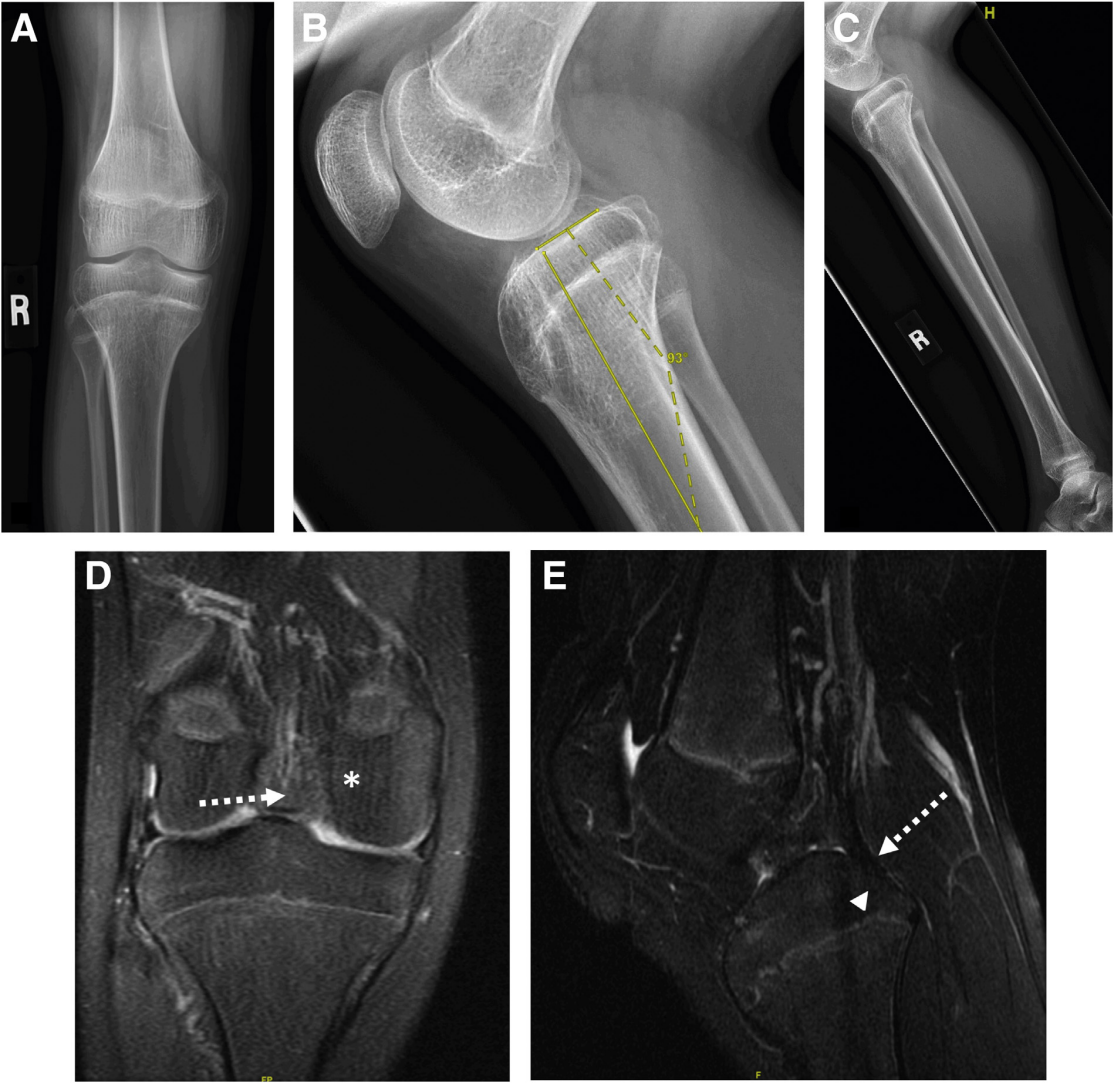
Example of reduced tibial slope. Anteroposterior (A) and lateral (B) radiographs of a right knee demonstrate neutral coronal alignment and tibial slope of −3°. A lateral radiograph of the tibia (C) shows no other deformity in the tibia. Corresponding coronal (D) and sagittal (E) magnetic resonance imaging in this patient shows absent posterior cruciate ligament fibers (dotted white arrows) at their origin on the lateral aspect of the medial femoral condyle (asterisk) and posterior tibial insertion (white arrowhead).
